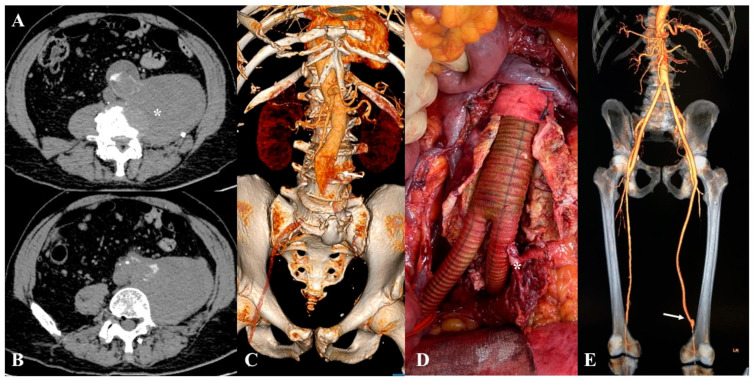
Preoperative computed tomography angiography (A,B) showing the infrarenal abdominal aortic aneurysm rupture into the left psoas muscle (asterisk). Volume rendering 3D reconstruction highlighted the site of rupture ((C), white arrow). Intraoperative finding (D) the final reconstruction after aortic graft replacement using a bifurcated graft with the inferior mesenteric artery transposition (asterisk). Follow-up computed tomography angiography at 48 months follow-up confirmed the absence of graft-related complication and the patency of the adjunctive above-the-knee femoro-popliteal reconstruction ((E), white arrow).
